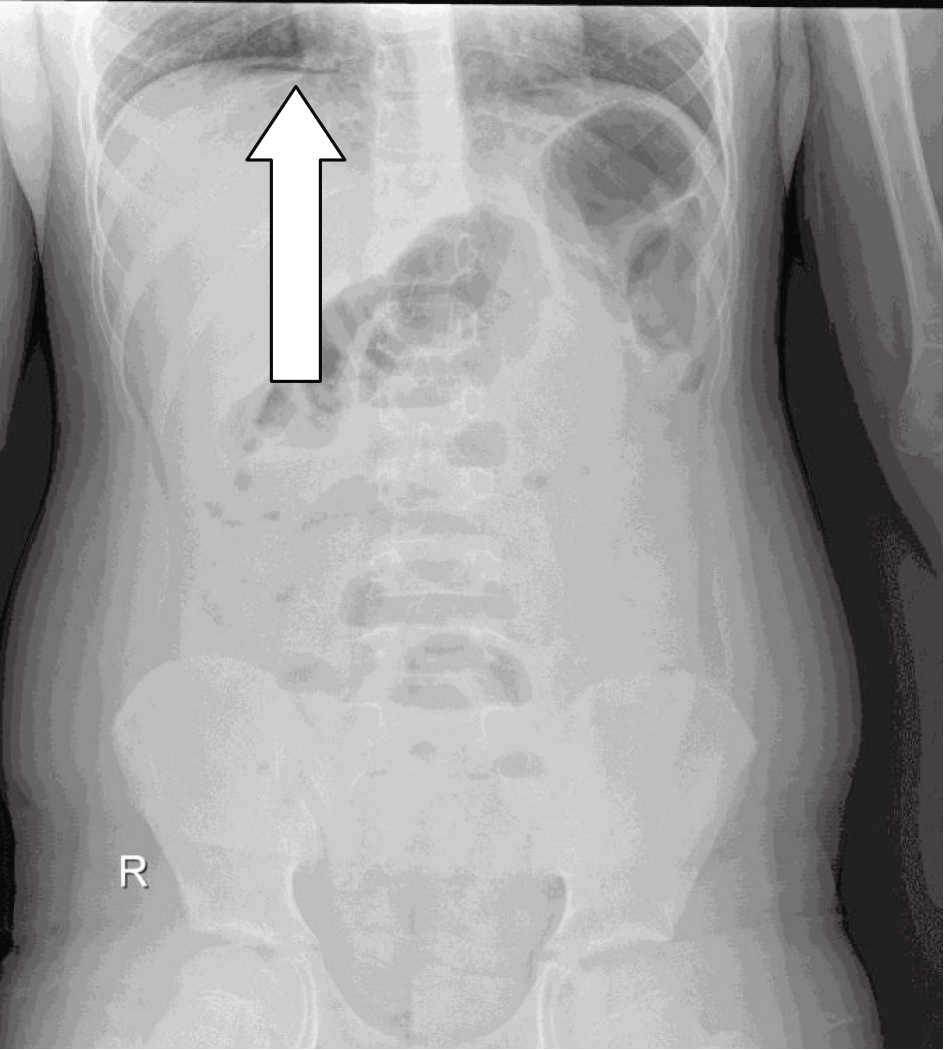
Plain abdominal erect radiograph showing subdiaphragmatic free air.
